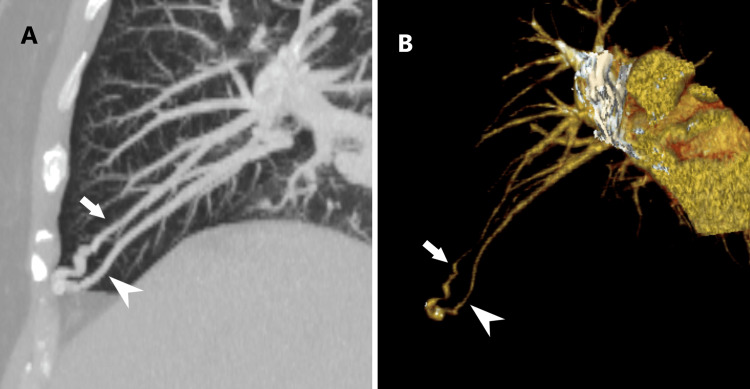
PAVM on CTPA (A) Sagittal oblique multiplanar reformatted maximum intensity projection image and (B) volume-rendered image from contrast-enhanced CT pulmonary angiography in a 53-year-old-woman shows the feeding artery (white arrow) and draining vein (white arrowhead) of a simple type pulmonary arteriovenous malformation, supplied by a pulmonary artery branch and draining into a pulmonary vein branch, respectively.
